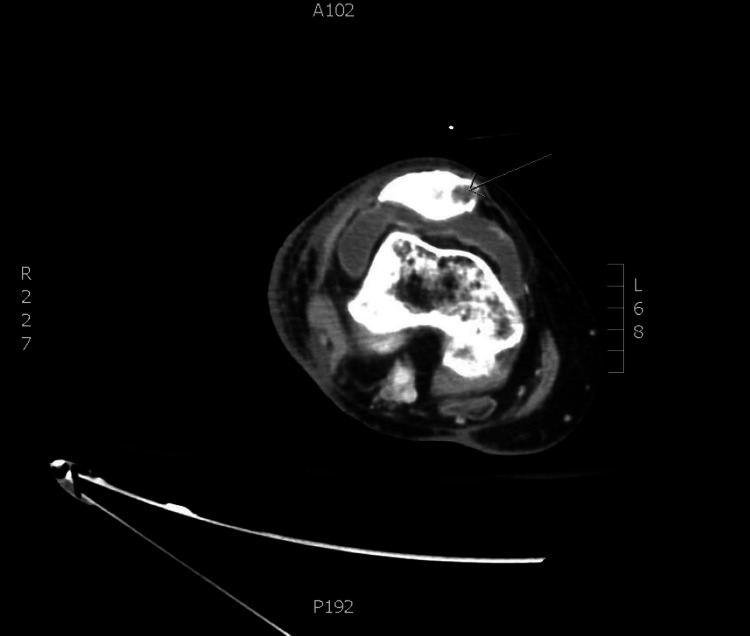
Osteomyelitis of the right knee A large right knee effusion with enhancing synovium and osteolytic nidus medial patella (arrow) is seen in a transverse view of the right knee on CT of the right femur #2 with IV contrast. Septic arthritis and osteomyelitis are confirmed at debridement surgery.
